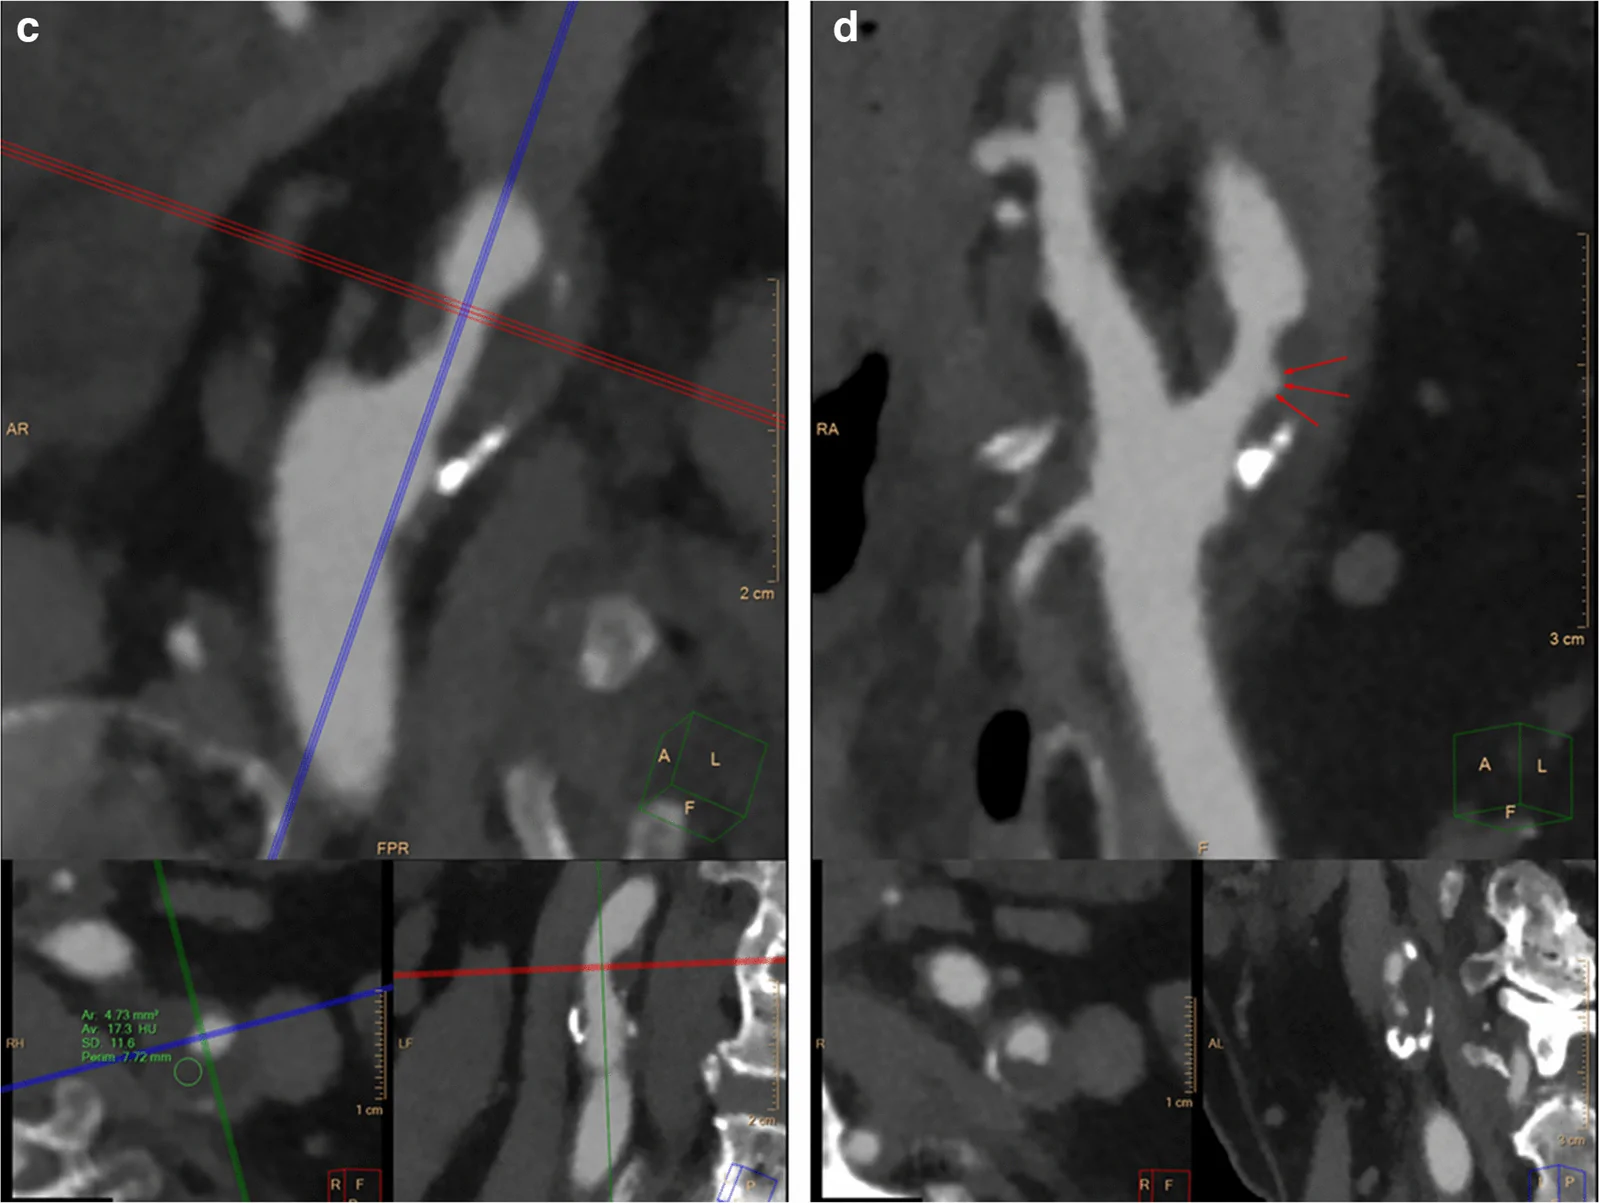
a–d Multiplanar CT reformats perpendicular to the residual lumen of the stenosed right internal carotid artery a–c with plaque density measurements (*green regions of interest*) in three consecutive axial slices, showing a dominantly lipid-rich plaque with an average density of 15–19 Hounsfield Units. d Contrast filling (*red arrows*) pointing at an exulceration within a dominantly lipid-rich plaque
